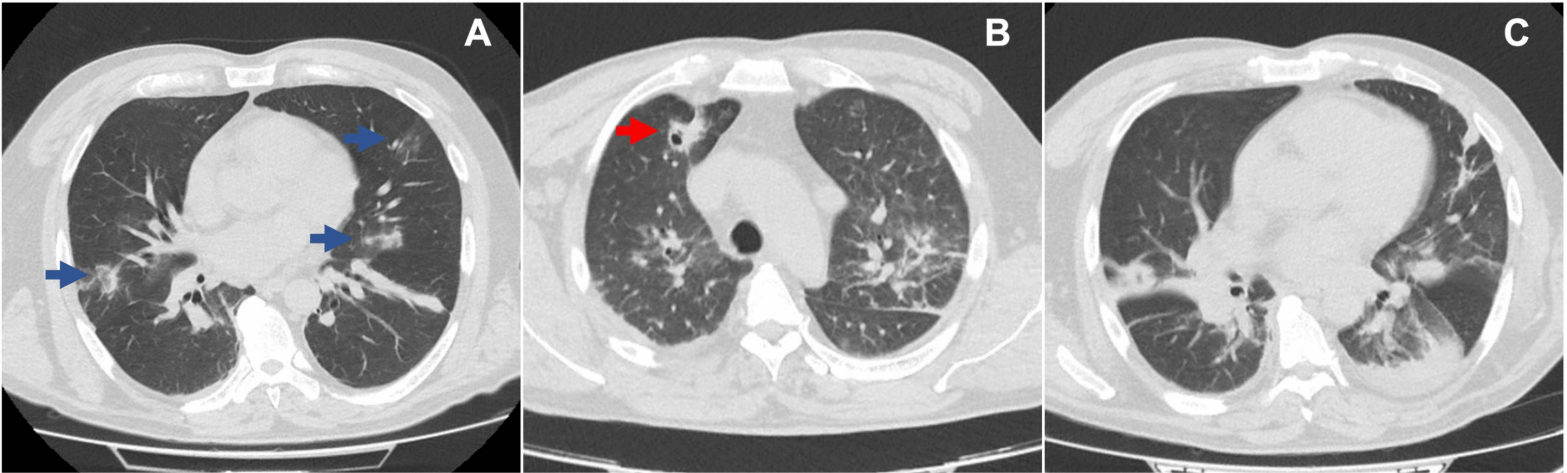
Computed tomographic pulmonary images. (A) On hospital admission, with small ground glass areas bilaterally (blue arrows). (B and C) On day three after hospital admission, showing bilateral areas of consolidation and cavitation in the right upper lobe (red arrow).
